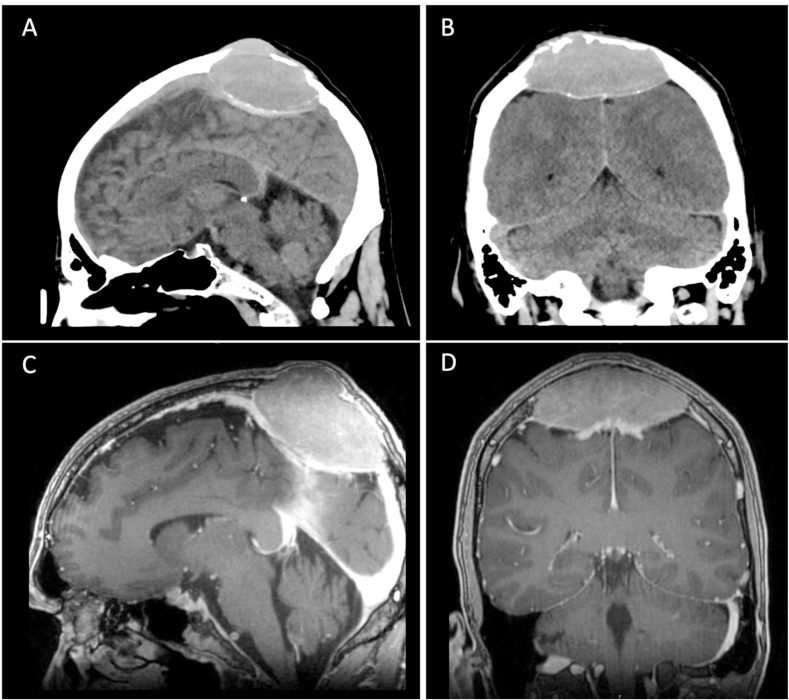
Preoperative imaging findings of the solitary intracranial plasmacytoma. A–B: Cranial CT (sagittal and coronal views) demonstrating a space-occupying lesion compressing the posterior third of the superior sagittal sinus (SSS), osteolysis of the skull, and expansion into the subcutaneous fatty tissue/into the cranial vault. C–D: T1-weighted MRI with contrast enhancement (CE-T1) (sagittal and coronal views) revealing homogeneous contrast uptake of the lesion, measuring 72 x 48 × 84 mm, suggestive of an osteomeningioma.
